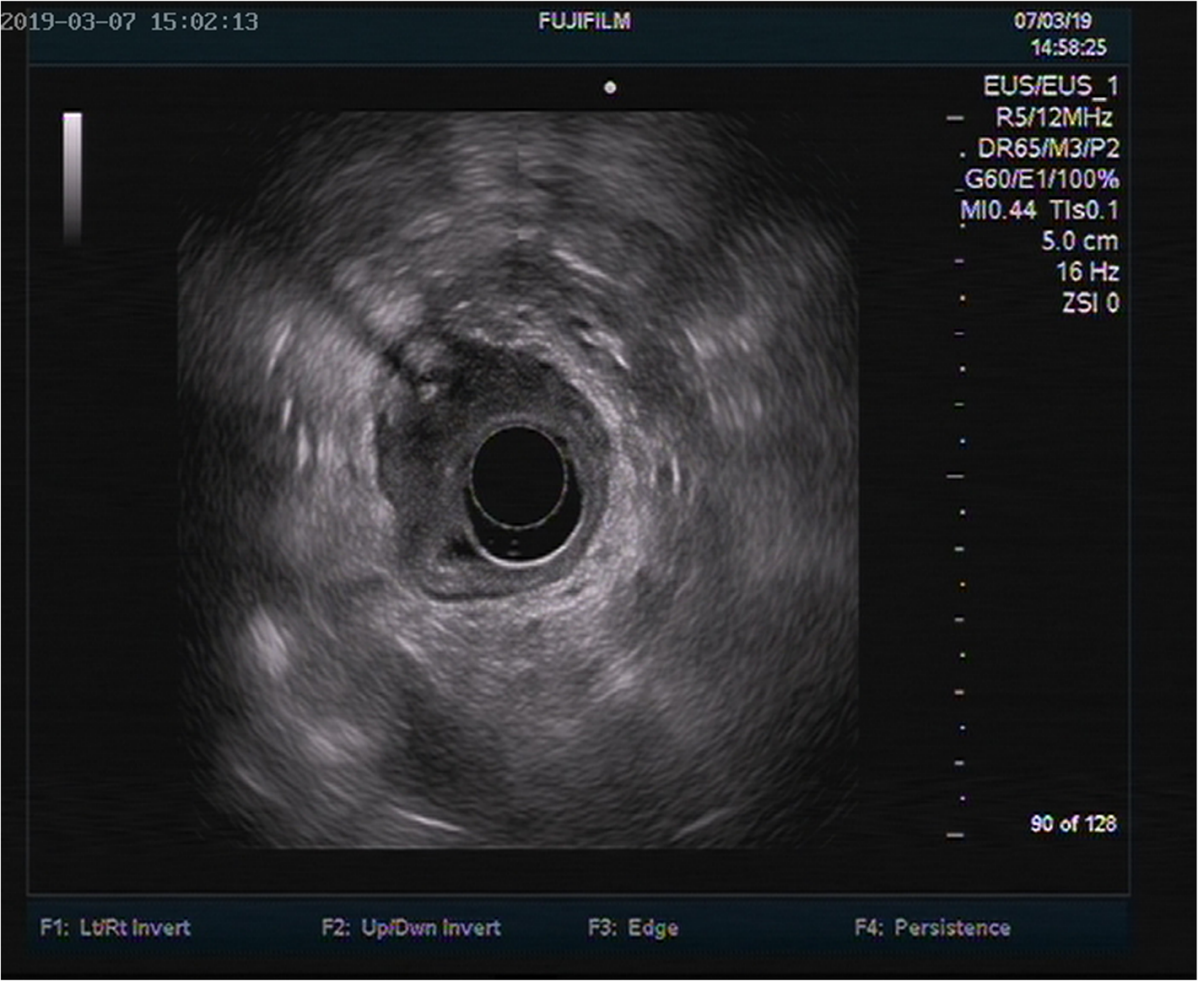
Ultrasound gastroscopy showed that the anterior wall of the duodenal bulb was obviously protruding into the cavity, a superficial ulcer was observed on the anterior wall, and white moss was observed on the uplift. Hyperechoic space was found in the deep submucosal layer of the local stomach, with a cross-section of about 0.1 × 0.1 cm^2^, accompanied by an acoustic shadow in the rear
